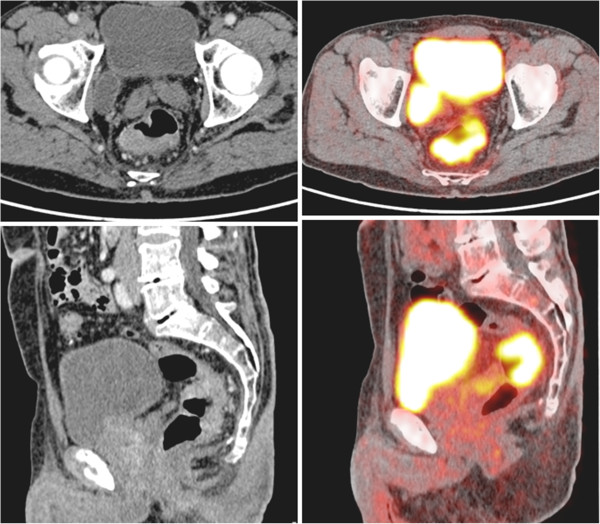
Radiological findings of the primary tumour. CT scan and Pet-scan of the rectal tumour prior to chemotherapy.
